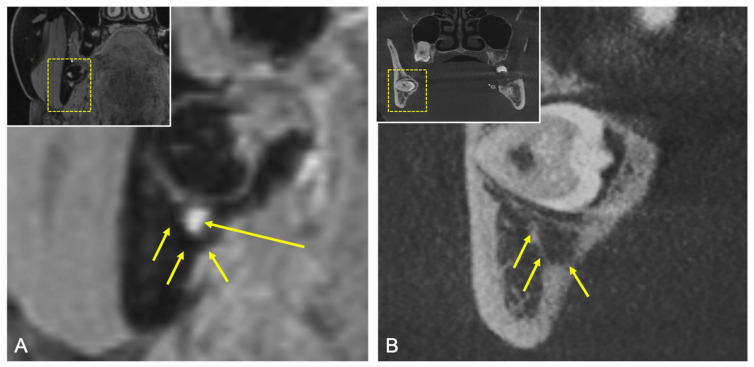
(A) Coronal reconstruction of the 3D-DESS MRI. For orientation, the dotted rectangles in the corner show the enlarged area. Short arrows showing the osseous boundaries of the IAC and the long arrow showing the MRI signal hyperintensities by the IAN’s tissue. (B) Coronal reconstruction of the CBCT. For orientation, the dotted rectangles in the corner show the enlarged area. Short arrows showing the osseous boundaries of the IAC.
